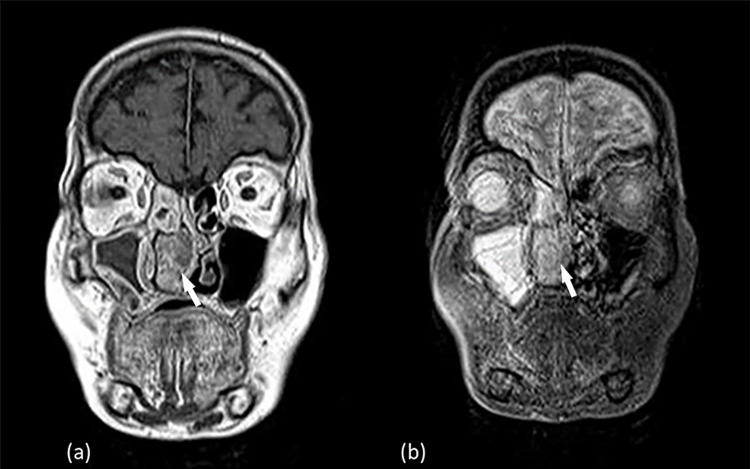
MRI coronal section. In the T1-weighted sequence the mass appears heterogeneous with low intensity signal (a). In the T2-weighted sequence is observed the heterogeneous mass with intermediate to high signal intensity (b).
